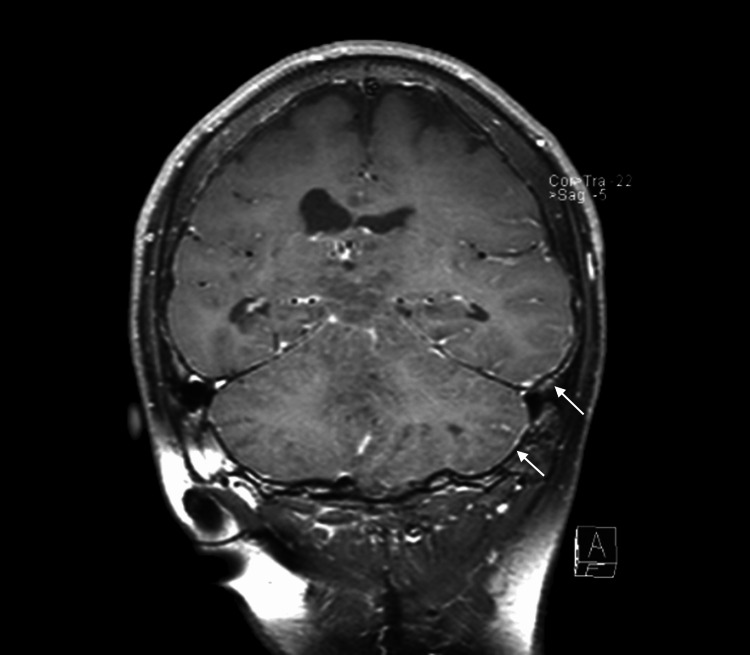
Contrast-enhanced T1-weighted coronal imaging of the brain showing diffuse subpial enhancement (arrows)
